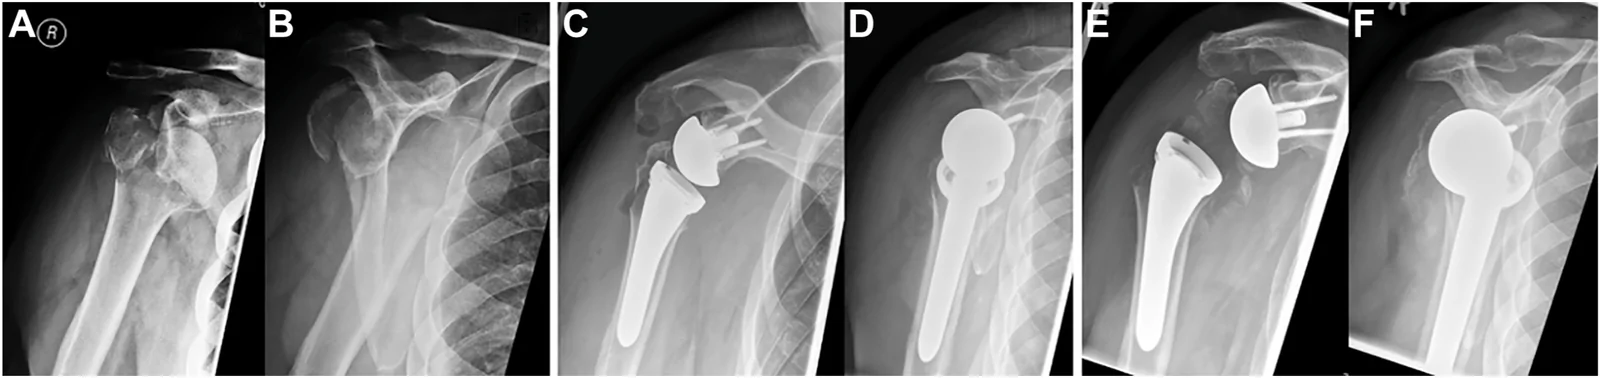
Radiographs of a 75-year-old male patient who sustained a PHF. The first images show the pre-operative fracture configuration. The patient was treated with rTSA. Subsequent images demonstrate post-operative positioning and later prosthetic dislocation, which occurred due to axillary nerve injury and resulting deltoid insufficiency. *PHF*, proximal humerus fracture; *rTSA*, reverse total shoulder arthroplasty.
